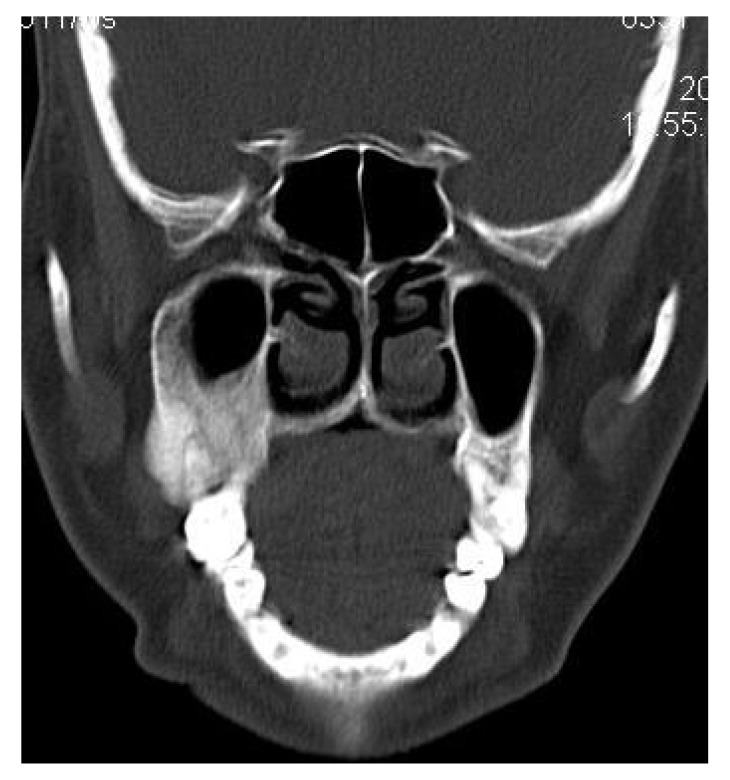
Coronal CT section revealing the involvement of left maxillary sinus and left maxillary alveolus.
